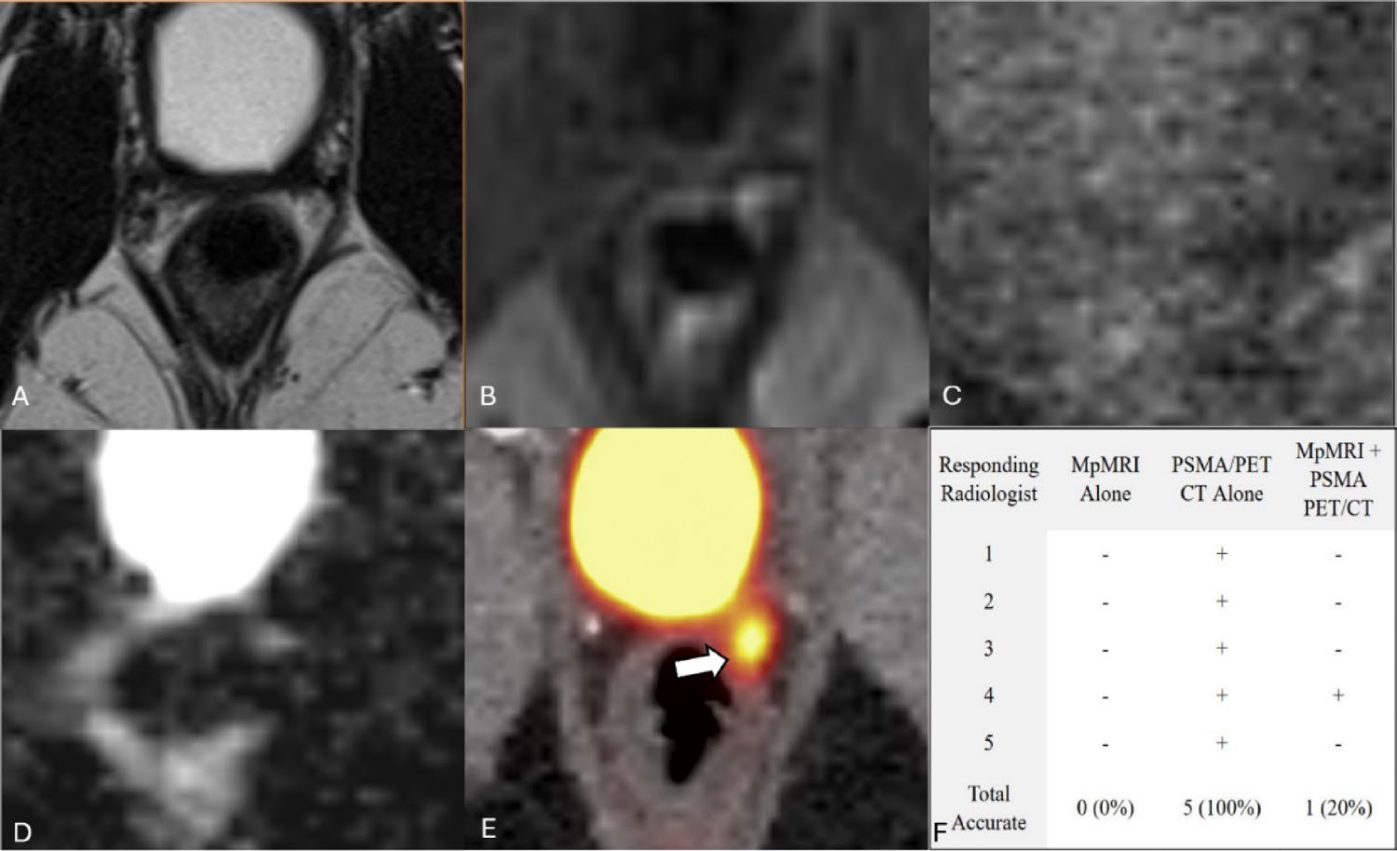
64-year-old patient with a left prostate bed lesion with focal uptake on PSMA PET/CT but no focal abnormalities on mpMRI. This case depicts a 64-year-old status-post prostatectomy indicated for a primary adenocarcinoma with a Gleason score of 4 + 3 = 7 with a T2b pathological stage. His PSA was measured to be 1.0 ng/mL at the time of imaging. MpMRI (A T2, B Arterial enhancement T1, C DWI, D ADC) shows no focal MRI abnormalities, but 18 F-piflufolastat PSMA PET/CT (E) shows a 5–10 mm area of focal uptake on in the left prostate bed (white arrow). MpMRI and PSMA PET/CT were performed 22 days apart. In this case, performance decreased with the combination of mpMRI and PSMA PET/CT when compared to PSMA PET/CT alone (F)
